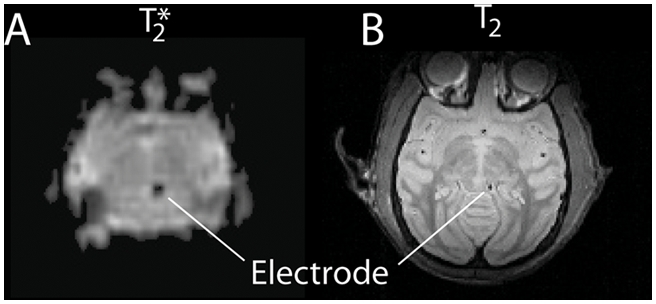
Artifact created by the microelectrode. T2* (a) - and T2-weighted images (b) show the extent of the artifact created by the microelectrode in the functional and anatomical images.
